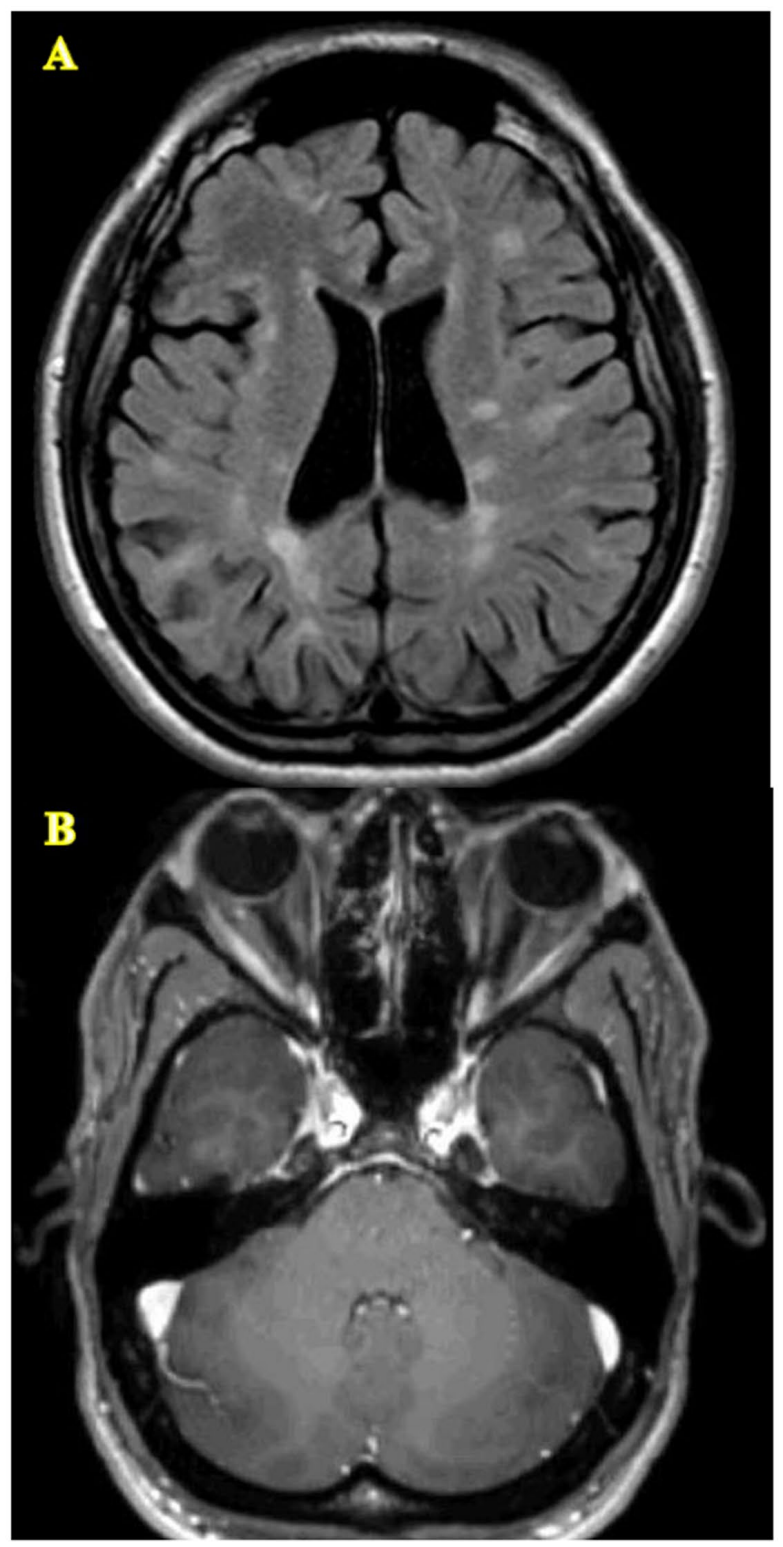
Lesions of the CNS characteristic of LHON-MS. (A) Diffuse T2/FLAIR signal abnormalities throughout the white matter meeting McDonald’s 2017 criteria. (B) Atrophy of the optic nerves.
